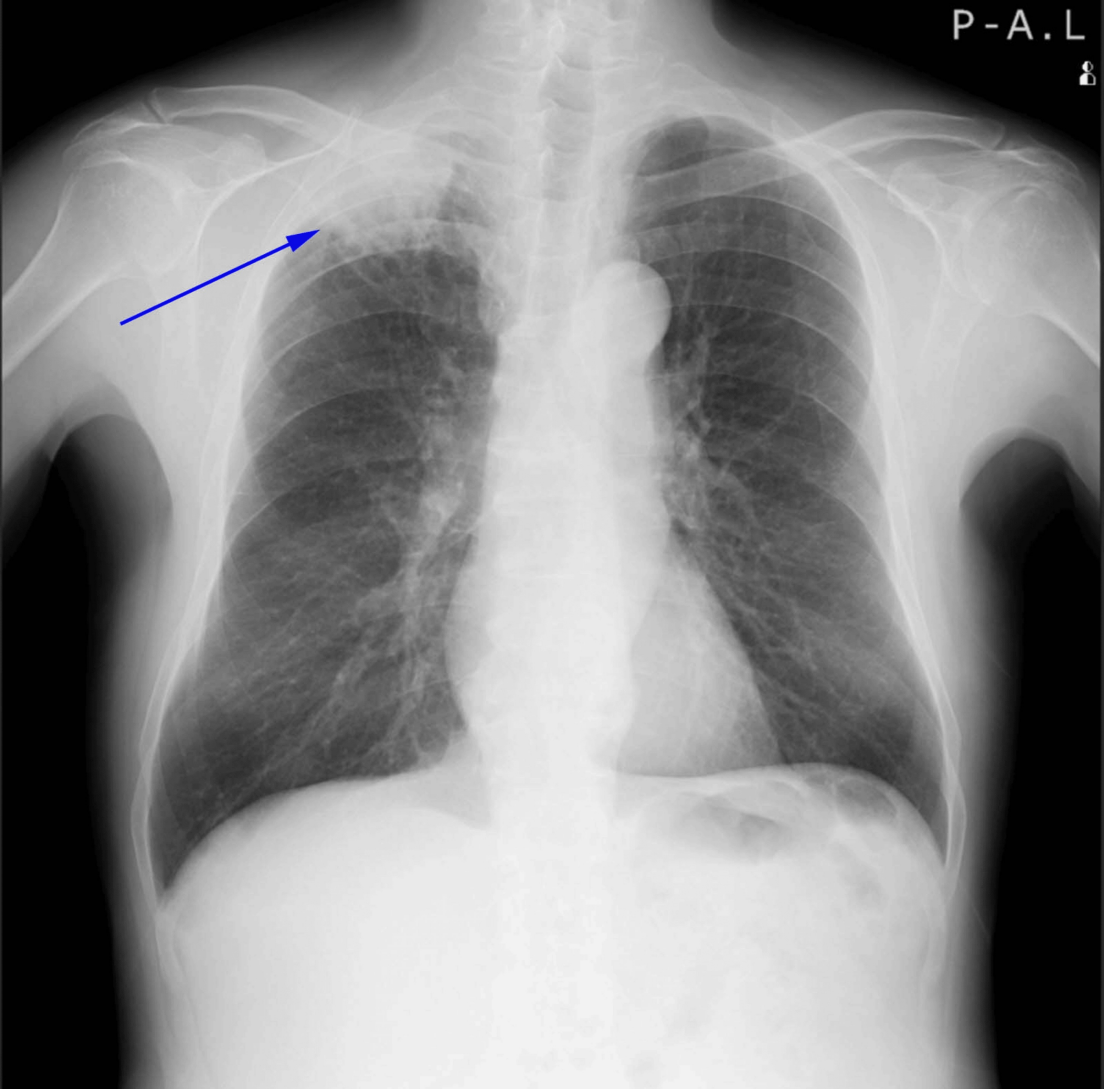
Routine chest X-ray on the day of admission showing a large mass (blue arrow) in the right upper lung
